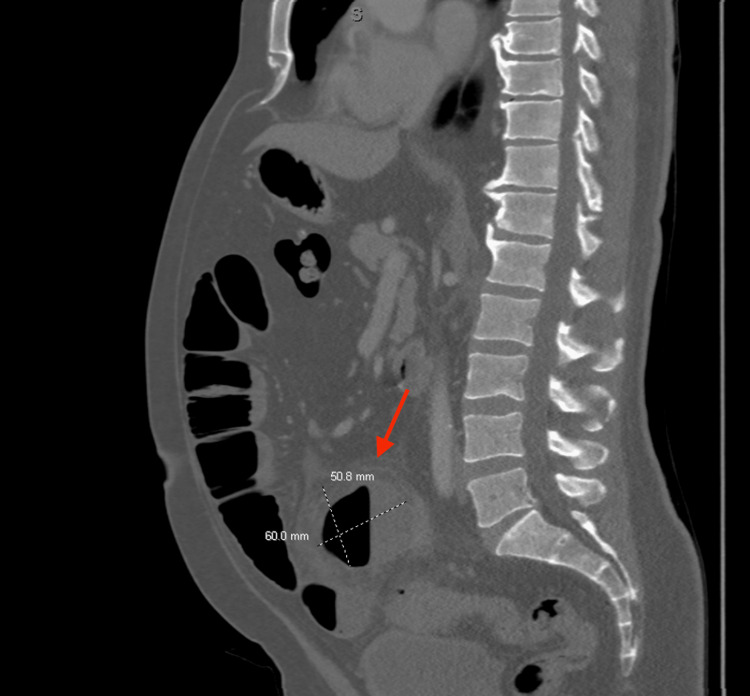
Sagittal View CT abdomen/pelvis with IV contrast CT: Computed Tomography; IV: Intravenous The CT image shows an abscess (50.8 x 60.0 x 60.0 mm, red arrow) with surrounding inflammation of the small bowel and sigmoid colon
